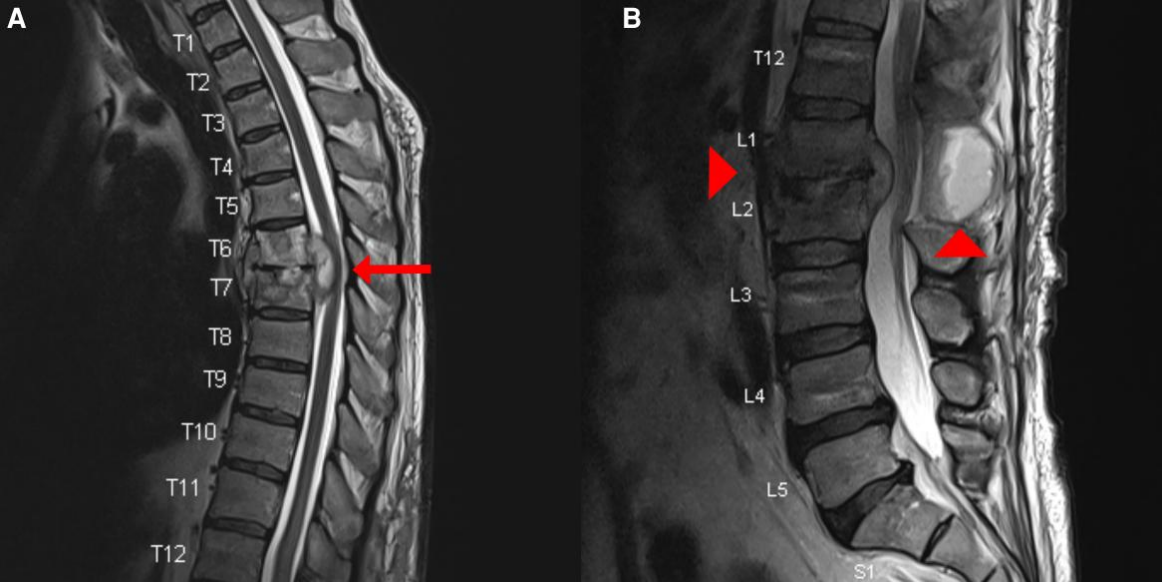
At the time of diagnosis, T2-weighted magnetic resonance imaging of the thoracic spine (*A*) and lumbar spine (*B*) in sagittal views. The arrow highlights spondylodiscitis of T6 and T7 with an associated epidural abscess causing mass effect on the spinal cord. The arrowheads highlight spondylodiscitis of L1 and L2 and a fluid collection at the laminectomy site.
